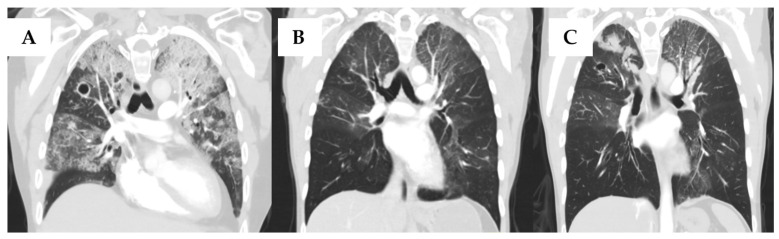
Computed tomographic (CT) scans of the lung of a 42-year-old female with nontuberculous mycobacterial infection (NTM) and non-*Aspergillus* invasive mold infection (NAMI) in a hematopoietic cell transplant recipient, who subsequently received bilateral lung transplantation. (A) Multifocal ground-glass opacities more prominent in the left lung; 1.5 cm cavitary lesion in the right upper lobe with centrilobular nodularity reported prior to NTM and NAMI therapy initiation. (B) Near-complete clearing of severe bilateral airspace consolidations after treatment of *Microascus* spp. and *Mycobacterium chimaera* infections. (C) Bilateral consolidative changes in the upper lobes, mostly at the time of diagnosis of *Aspergillus calidoustus* infection.
